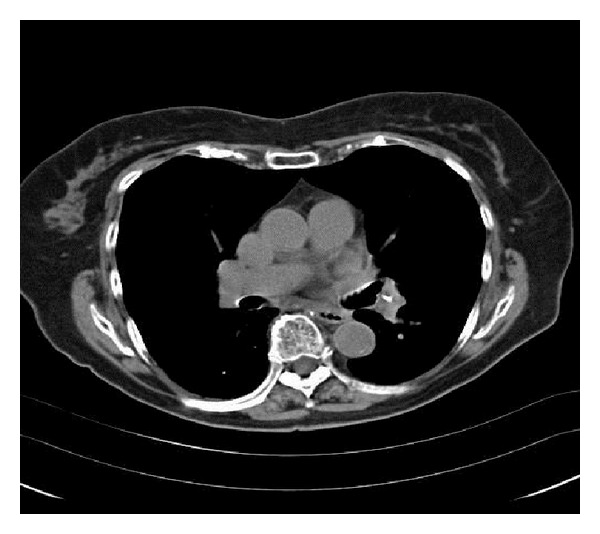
Computed tomography of chest without contrast depicting a filling defect in the branch of left pulmonary artery.
